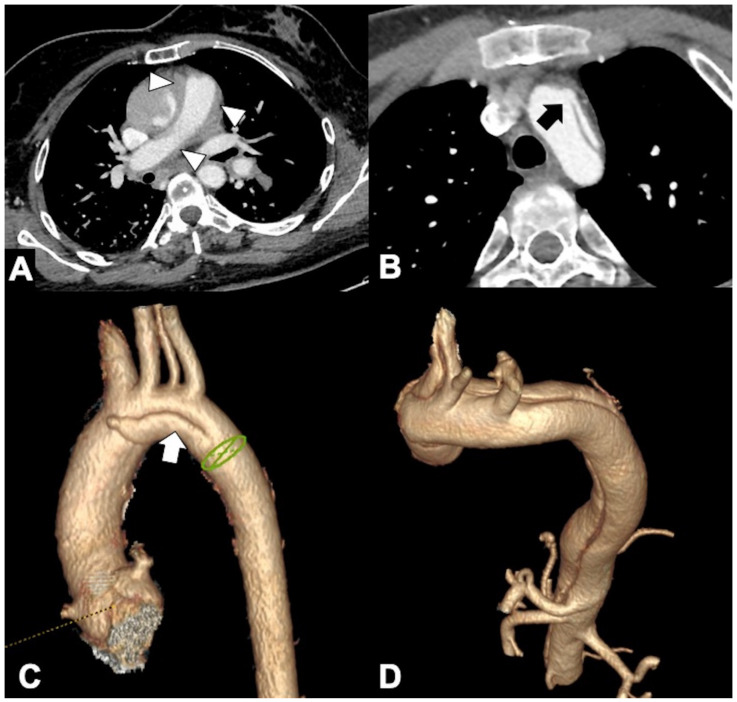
(A) Axial CTA image demonstrates thickening of the main pulmonary arterial wall (arrowheads) indicating IMH of the PA in ruptured Type A AD. (B) Axial CTA image shows entry tear (arrow) in the arch convexity in a 53-year-old man with severe chest pain. (C) 3D-VR parasagittal reconstruction image shows localized 4-vessels arch dissection (arrow) without supra-aortic trunks involvement. (D) 3D-VR parasagittal reconstruction image shows a non-A-non-B dissection with arch-entry and anterograde descending aorta involvement.
